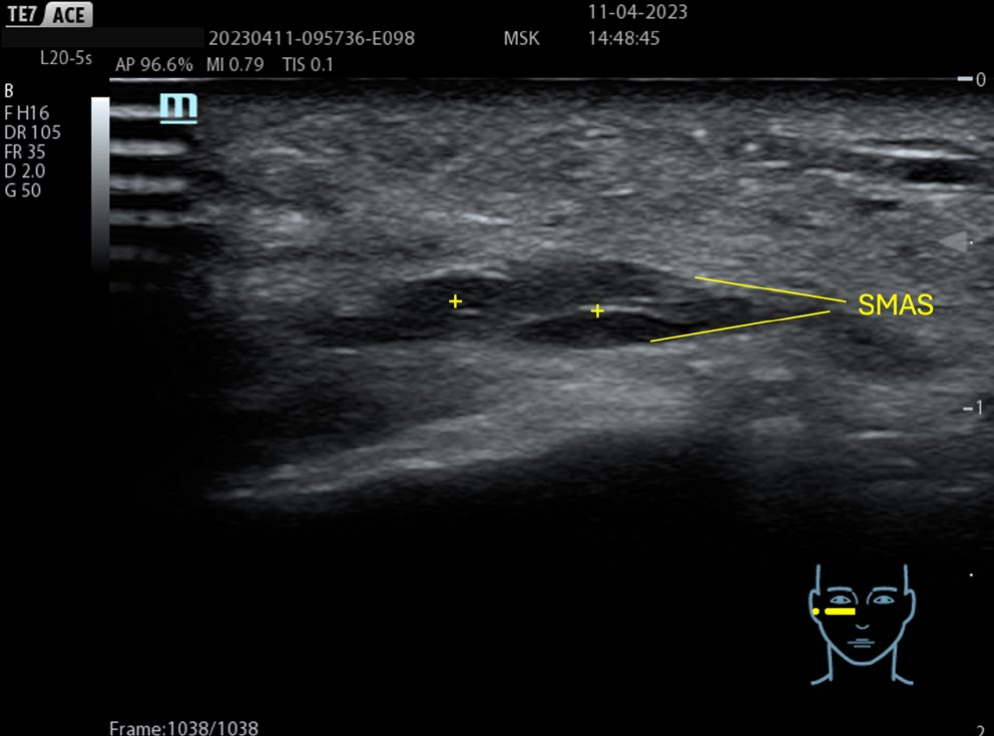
HA deposition (+) within the SMAS is possible when the treatment is performed without ultrasound guidance.
